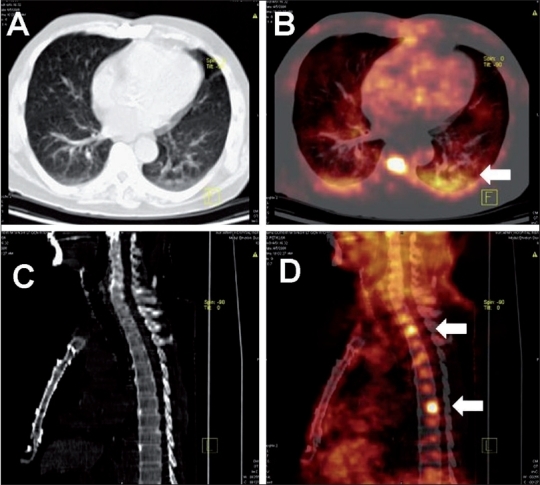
Pulmonary and spinal tuberculosis. Axial CT chest (A), axial PET/CT (B), sagittal reconstructed spine (C), and sagittal PET/ CT (D) images in a patient with FUO show FDG-avid reticulonodular lesions in the left lung with associated FDG-avid foci in two dorsal vertebrae (arrows), with no bony lesion seen in the corresponding CT images. The patient responded well to empirical antituberculous therapy
